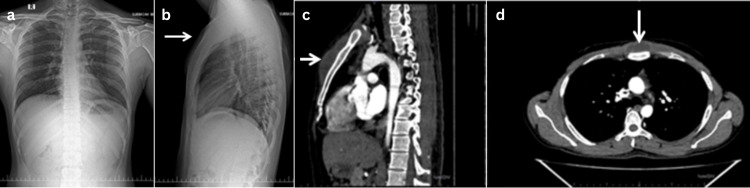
Extraspinal case 2: sternal TB (a, b) Chest X-ray posteroanterior (PA) view and lateral views, respectively, appears essentially normal. (b) The arrow in the lateral view indicates subtle soft tissue swelling. (c, d) High-resolution computed tomography (HRCT) of the thorax in sagittal and coronal views, respectively, arrow mark showing thick walled hypodense intramuscular collections with enhancing walls anterior to the manubrium, body of sternum, and left parasternal region, suggestive of an abscess. Data shown are representative radiographic findings from a single patient case (extraspinal case 2 - sternal TB). Original images obtained by the authors at Subbaiah Institute of Medical Sciences, Shivamogga.
